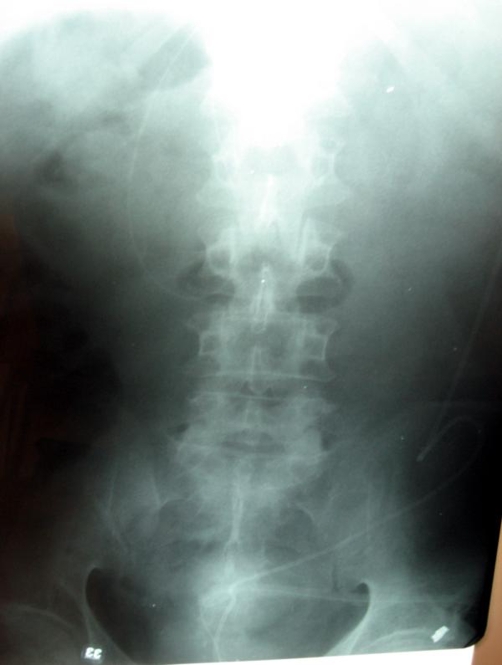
Plain abdominal radiography showing the ventriculoperitoneal shunt catheter within the colonic lumen.
